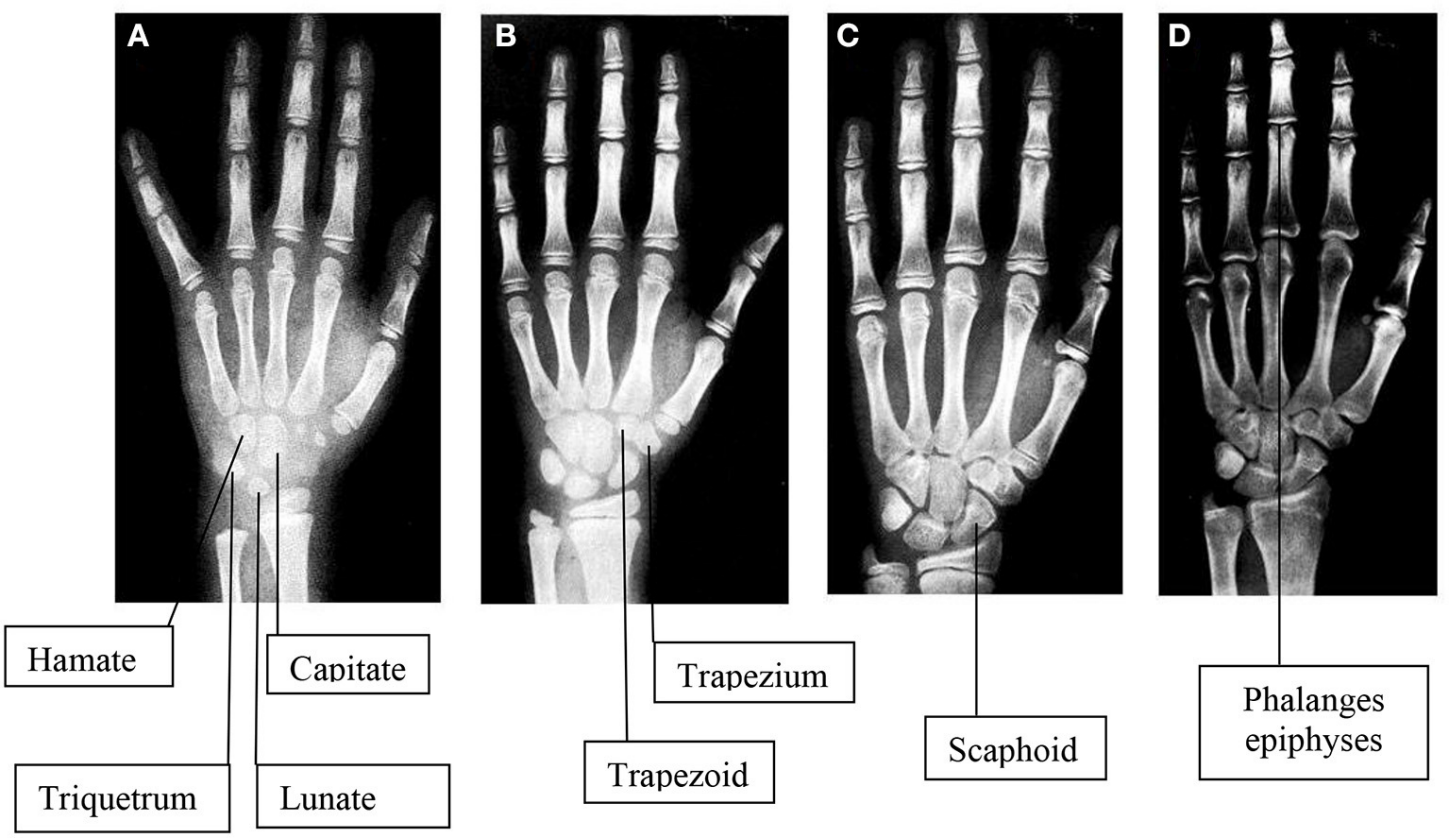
Images of hand and wrist x-rays in four female subjects compatible with physiological skeletal maturation in different ages: A (4 years), B (8 years), C (12 years), D (16 years). To note, usually the first ossification center to appear is in the contest of Capitate and Hamate at the second month in females and around the fourth month in males. Then the remaining centers appear, including Triquetrum at 2 years in females and 3 years in males, Lunate at 3 years in females and 4 years in males, Trapezium at 3 years in females and 4 years in males, Trapezoid at 4 years in females and 6 years in males, Scaphoid at 4 years in females and 6 years in males, Pisiform at 9 years in females and 12 years in males [77].
